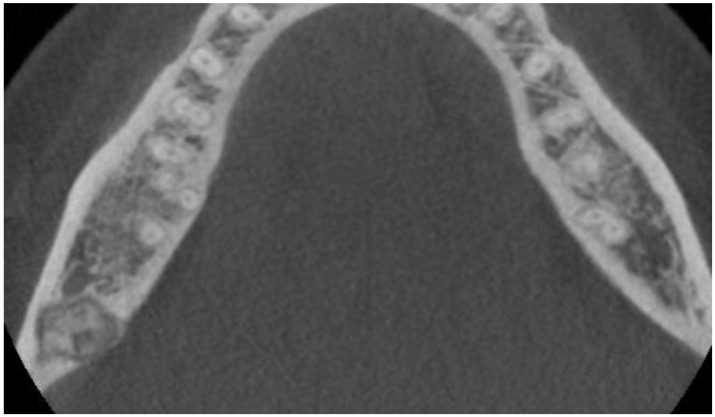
CBCT axial view with COD spread towards the lingual cortical plate.
